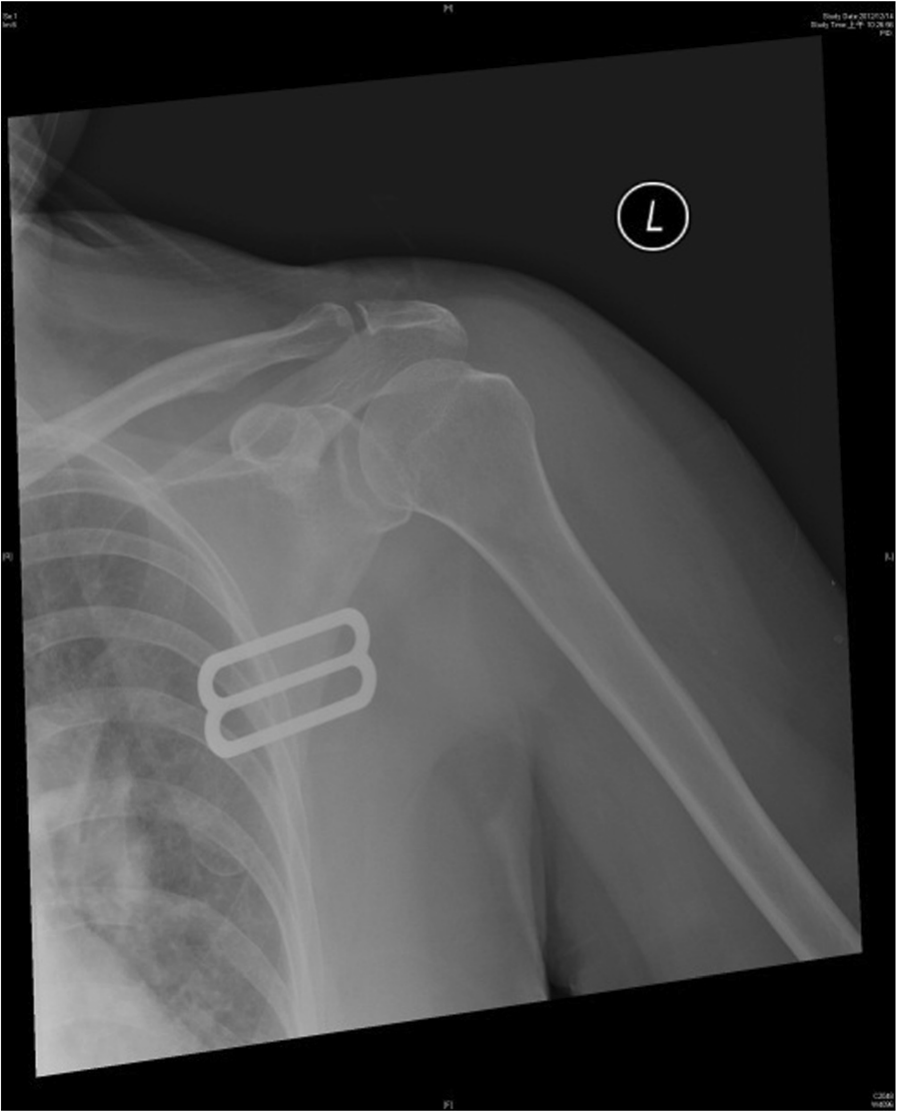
Left shoulder radiograph showing successful closed reduction.
